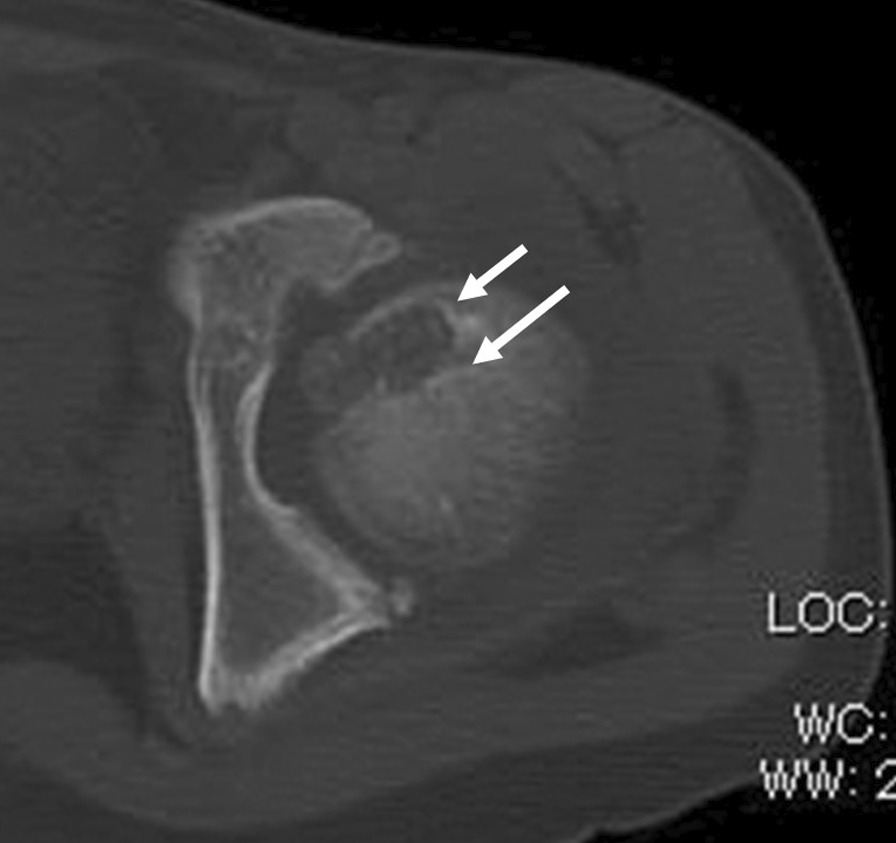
Preoperative computed tomography (CT) image: Case 2. CT images revealing a lytic lesion with a sclerotic rim located in the anteromedial side of the femoral head. The white arrow indicates the direction in which the osteotome was driven
